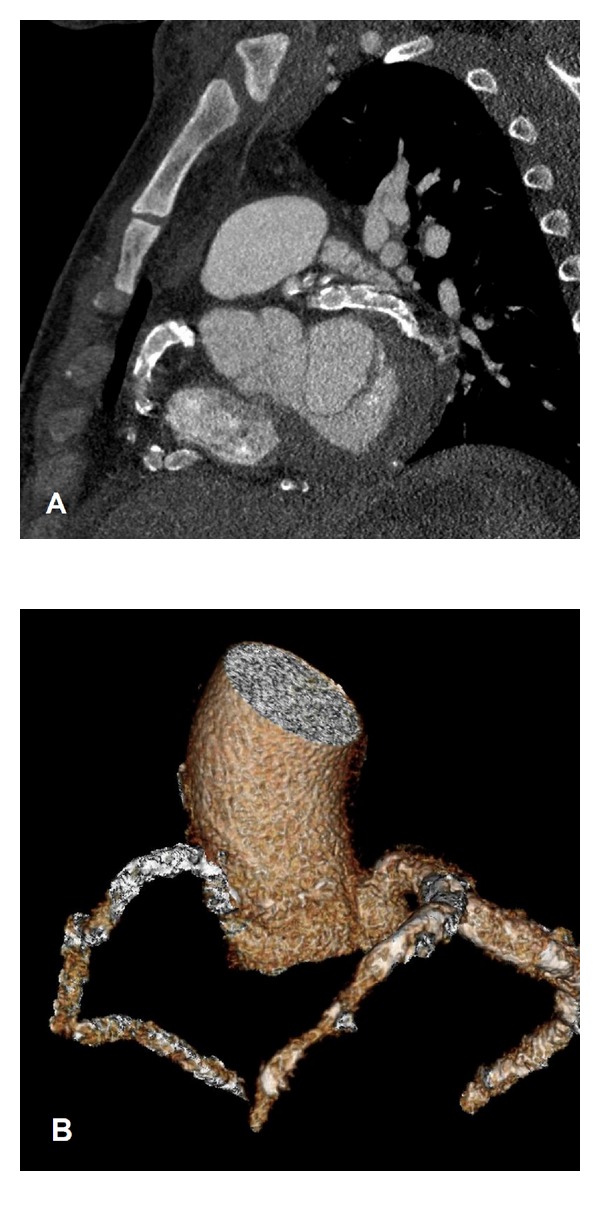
Dual source computed tomography showing (A) diffuse calcification in RCA and LCx and (B) 3-dimensional volume rendering image demonstrating coronary arteries ectasia.
